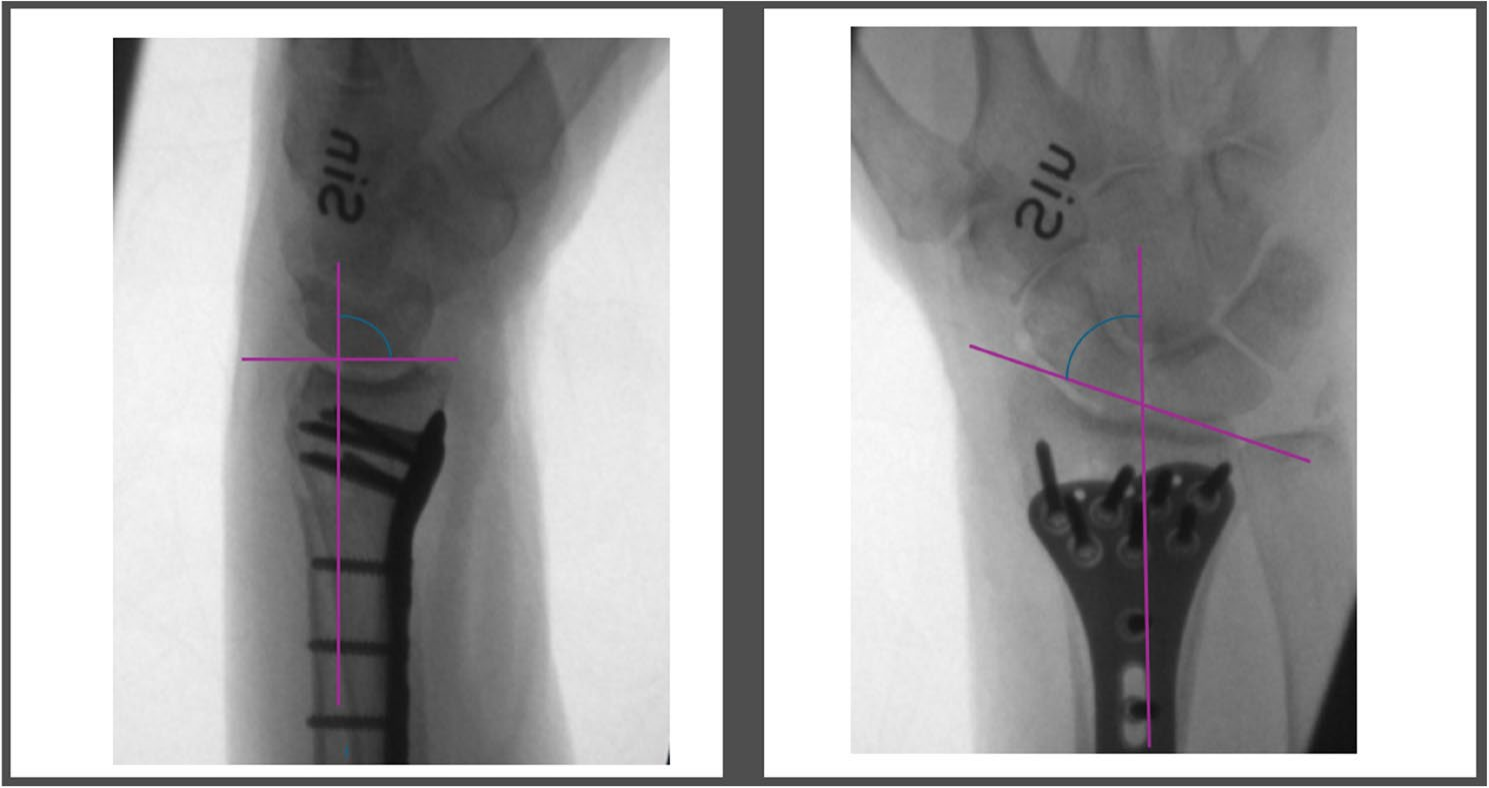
Assessment of dorsal and radial tilt on lateral and anteroposterior radiographs [14]
